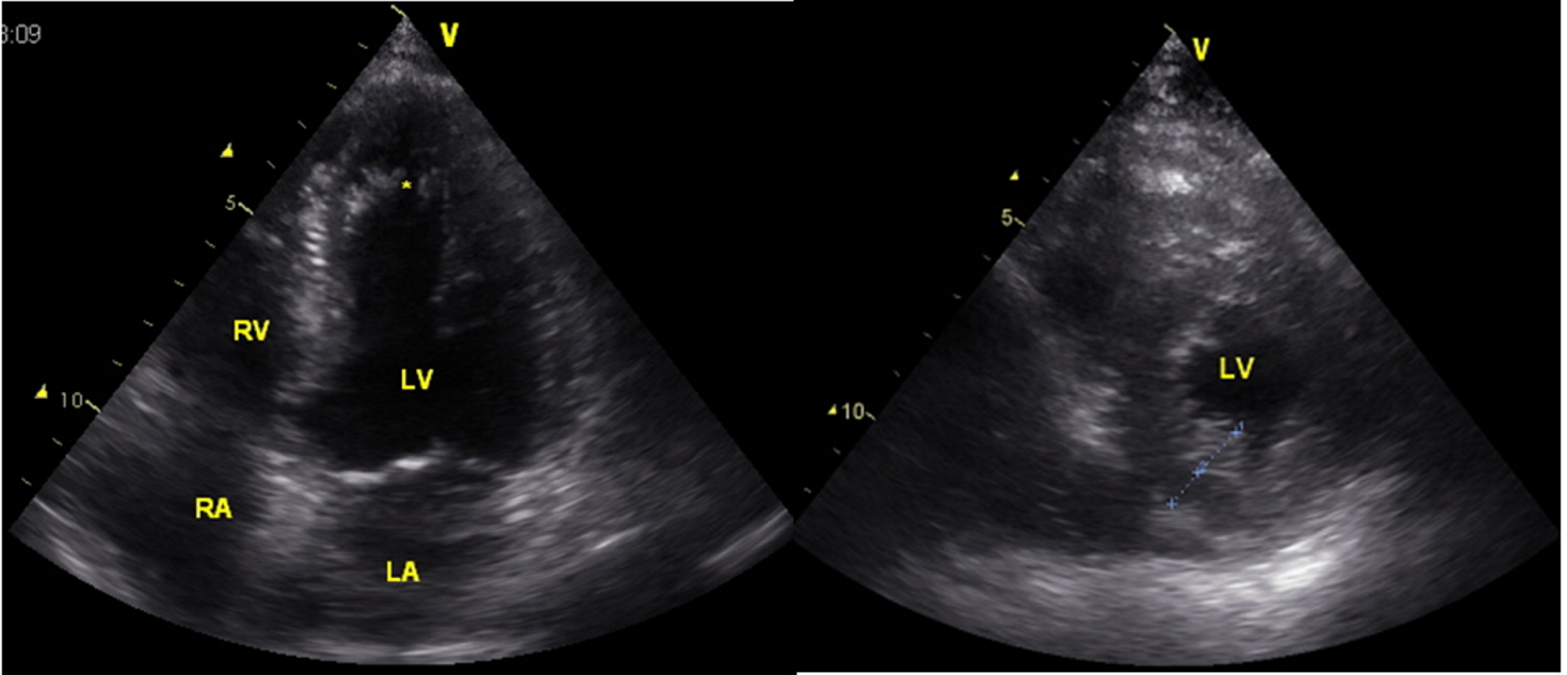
Transthoracic echocardiography 4-chamber view showing left ventricular hypertrabeculation (LVHT)/left ventricular noncompaction cardiomyopathy (LVNC) of the left ventricular apex (*) and the adjacent septal and lateral myocardium (left panel). In the parasternal axial view a two-layered structure of the myocardium (inner layer is echodens and hypertrabeculated and the outer layer is homogenous) distal to the mitral leaflet is visible (right panel).
